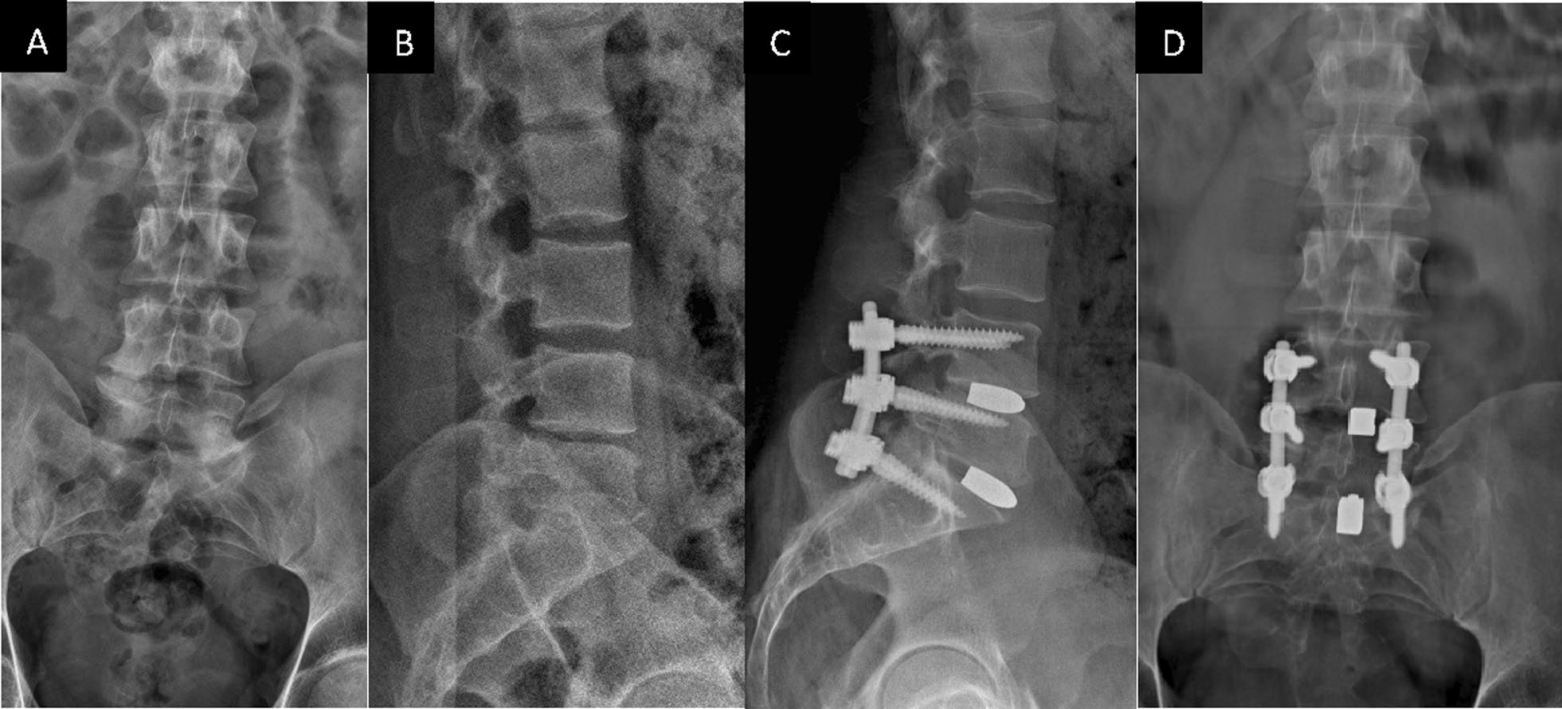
Lumbosacral spine X-ray: A pre-op AP view, B pre-op lateral view (PT 18°, SS 16°, L4–S1 lordosis 13°, global lumbar lordosis 24°), C post-op lateral view (PT 7°, SS 27°, L4–S1 lordosis 29°, global lumbar lordosis 41°), D post-op AP view
